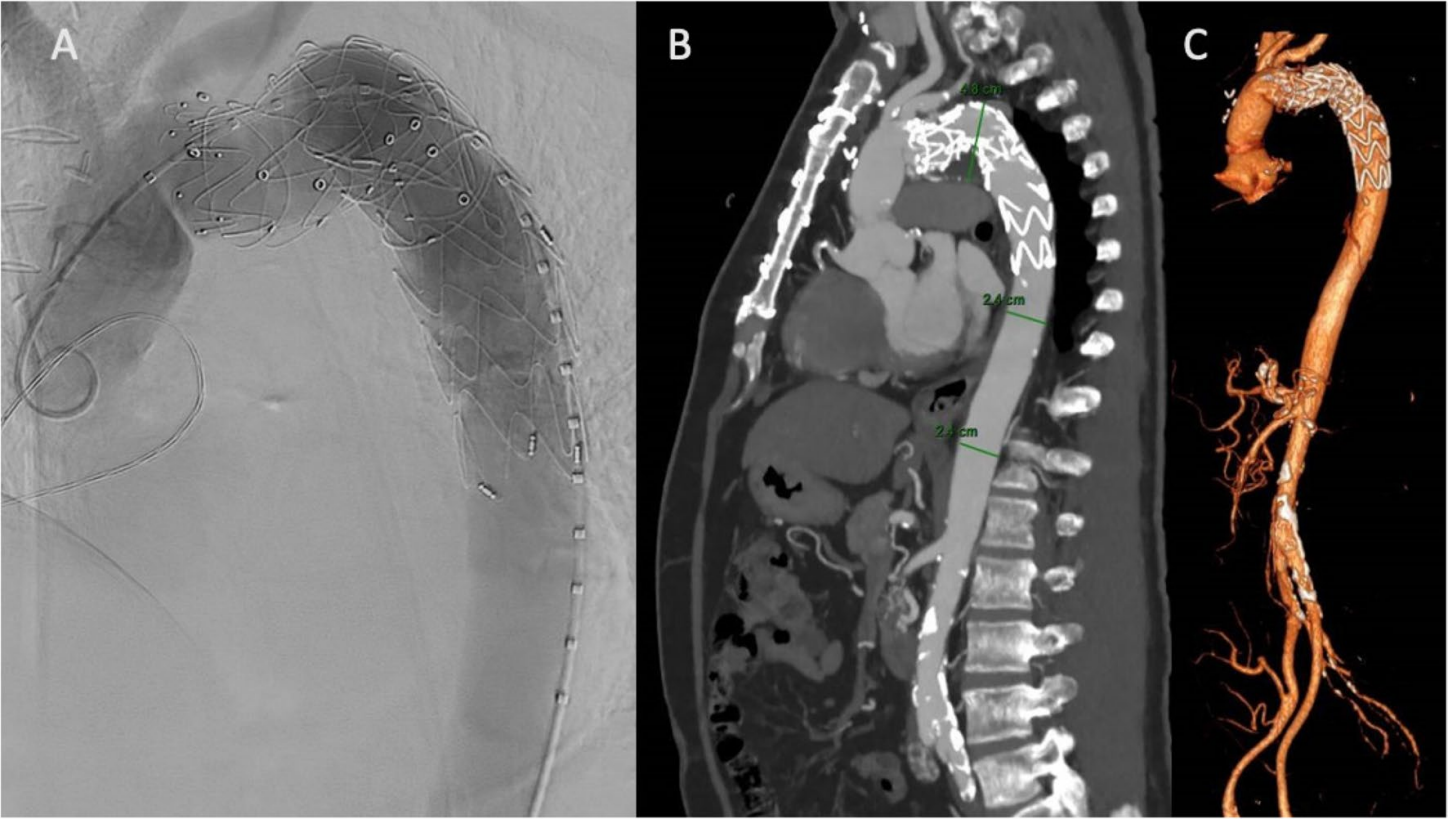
Results of TEVAR in the same patient as Fig. 2. A Angiographic control. B and C Post-operative CT-scan. *TEVAR, thoracic endovascular aortic repair; CT, computed tomography*
